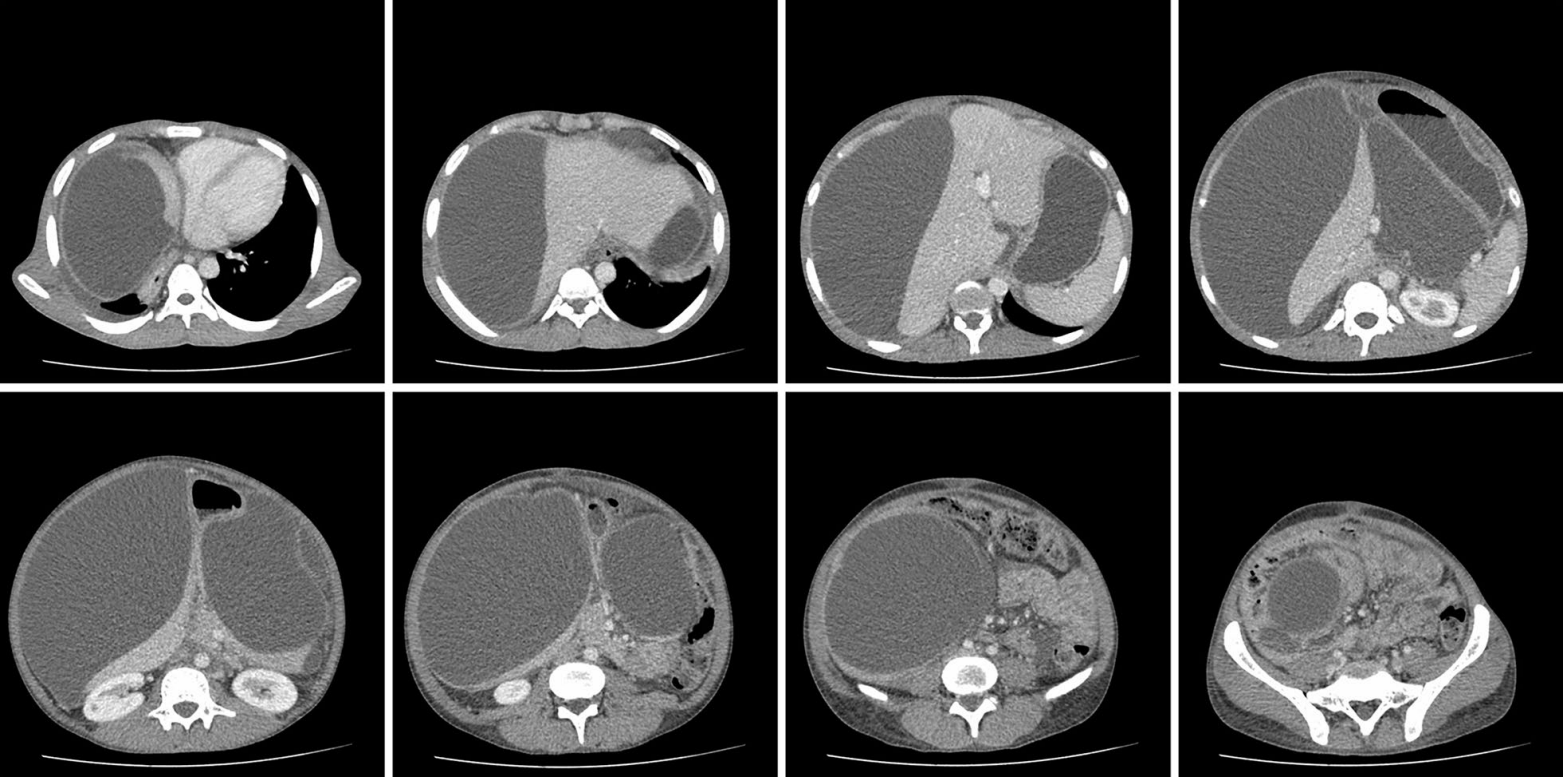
Contrast enhanced CT scan of the abdomen—axial view—large multilocular cystic lesion occupying almost the entire abdominal cavity
